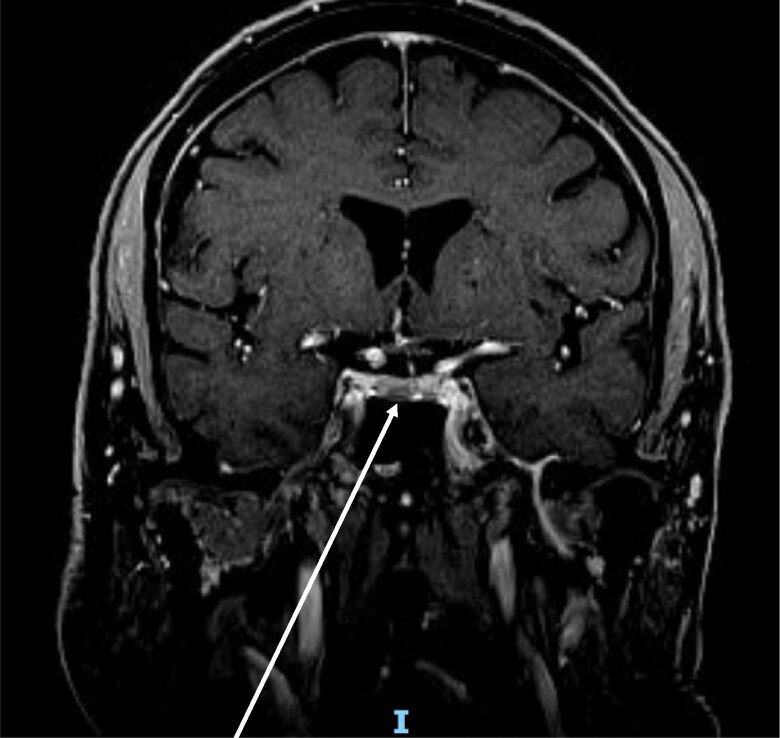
Gadolinium-enhanced pituitary magnetic resonance imaging (coronal view; T2).
